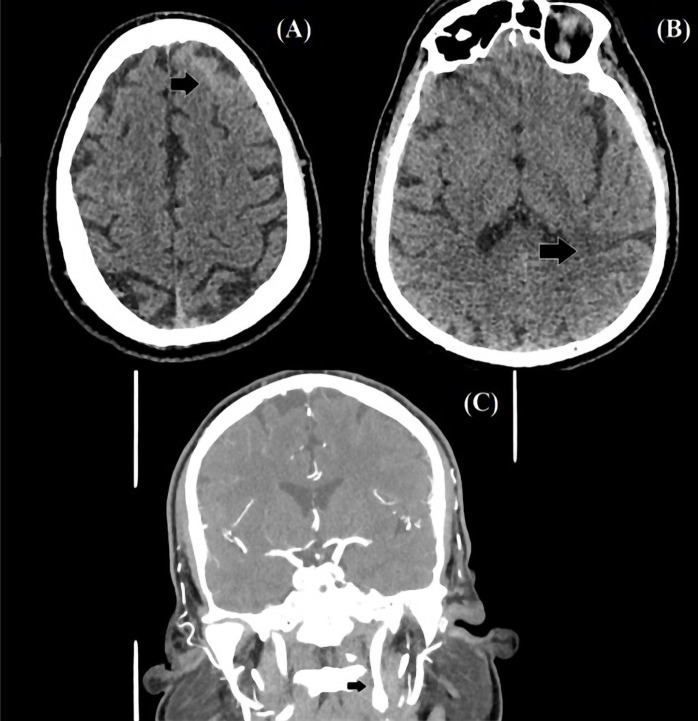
CT scan of the brain. Axial cross-section images showing the subarachnoid hemorrhage in the left frontal lobe (A) and the ischemic lesion in the left temporoparietal region (B). CT angiography, coronal cross-section, revealing a small filling defect in the cervical segment of the left internal carotid artery (C).
